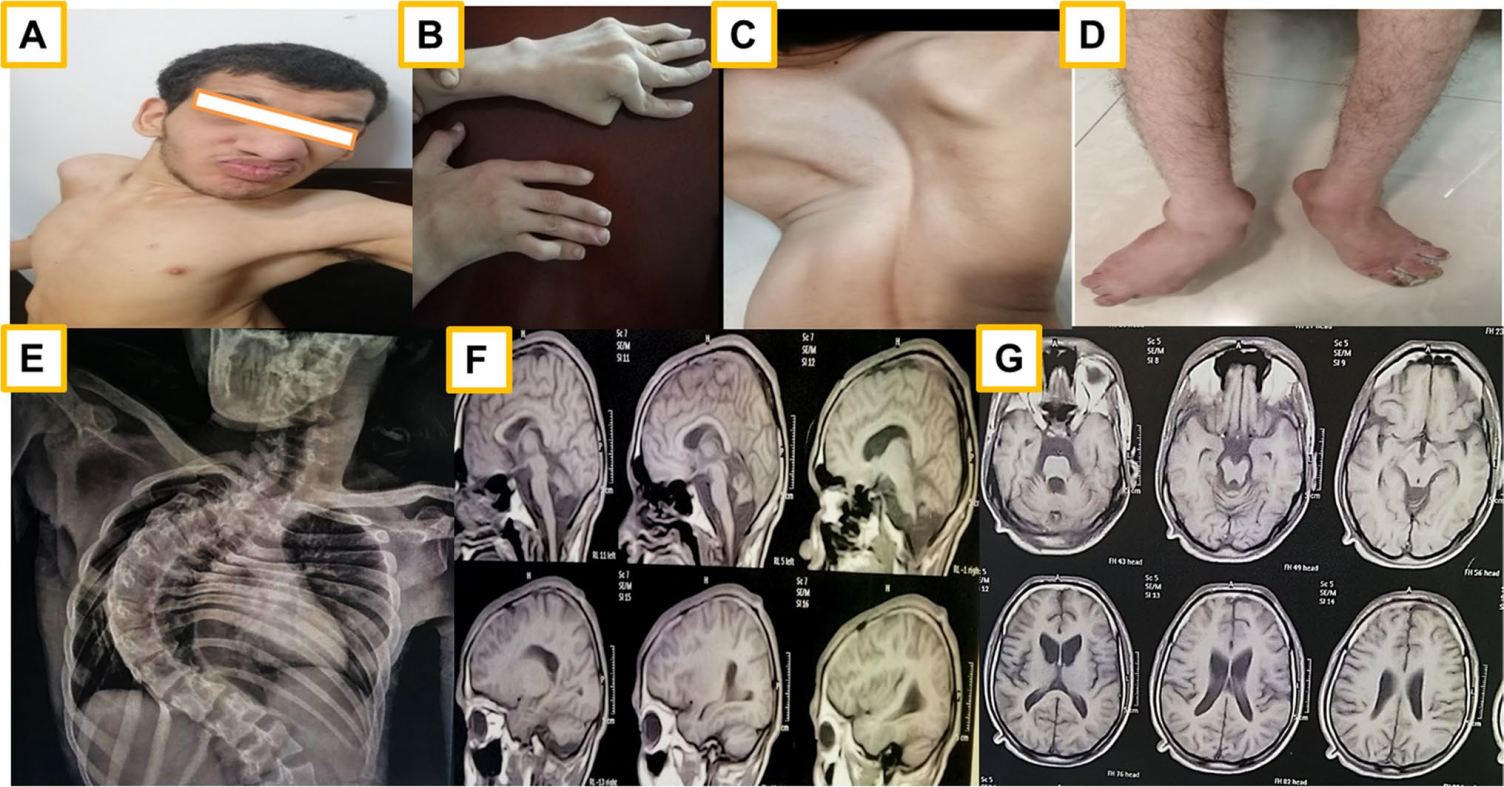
Patient 3—phenotypic dysmorphic features, scoliosis, skeletal deformities, and fungal feet infection. X-ray chest PA view showing severe scoliosis and ribs crowding (E), MRI brain (F, G) showed cerebellar atrophy
